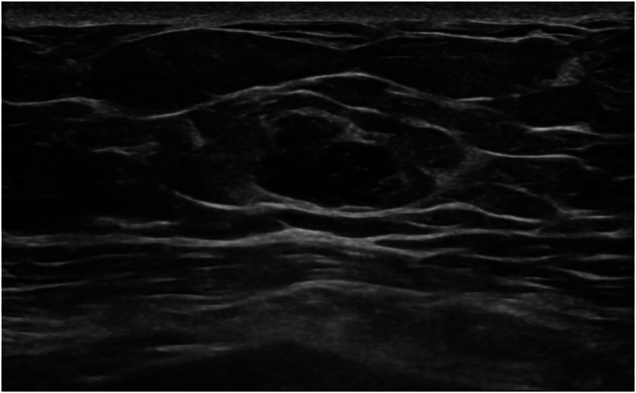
Breast ultrasound showing a 13mm ill-defined hypoechoic mass
